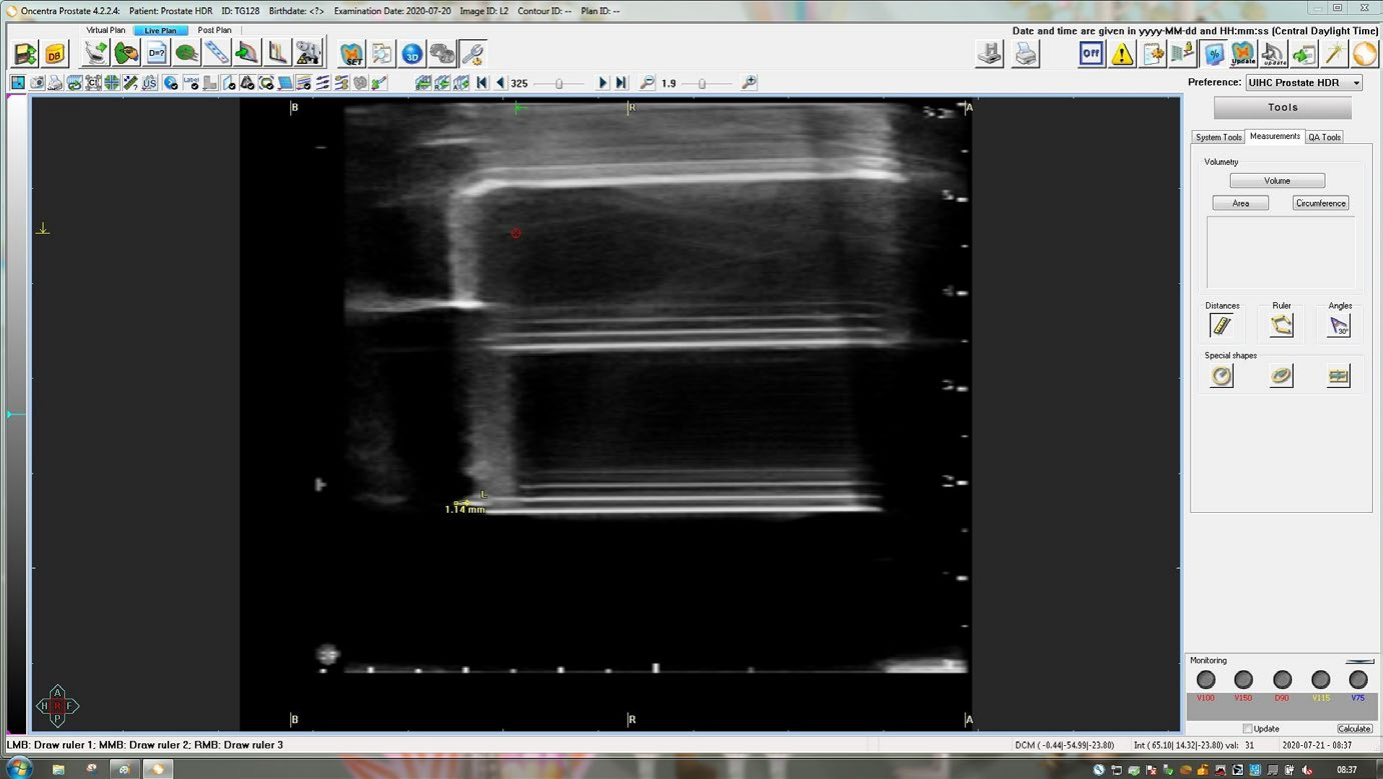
Needle tip displacement measurement from a reconstructed needle in the TPS (Oncentra Prostate) to the ultrasound marker imaged from a sagittal scan. Displacement was measured from the discernible tip of the needle to the center of the marker
